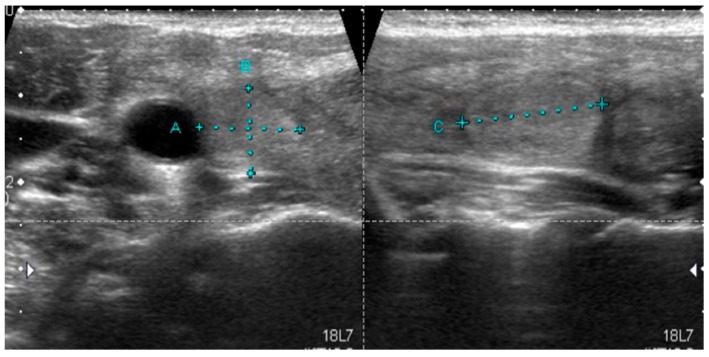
Typical sonographic pattern of *RAS*-positive NIFTP, normoechoic lesion with well-defined margins and without calcifications.
